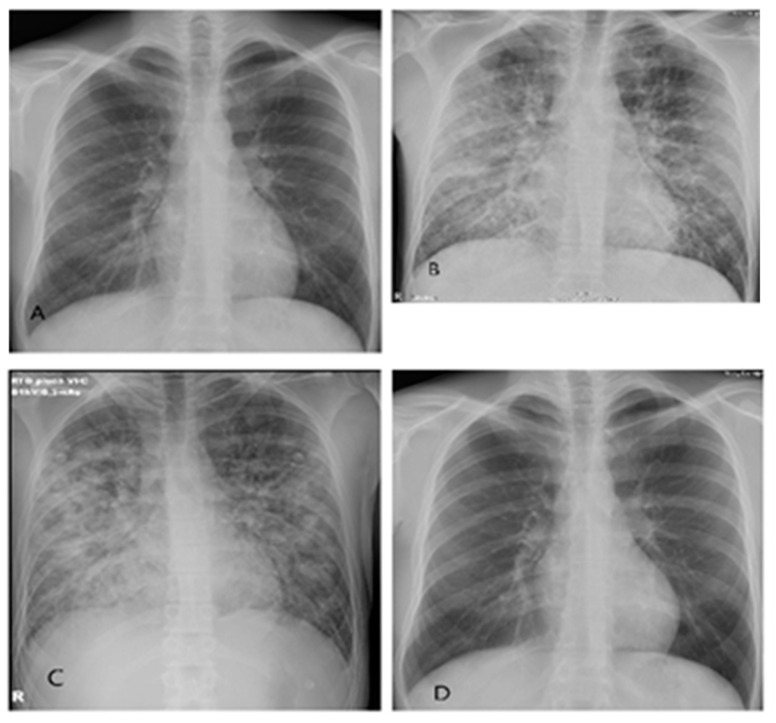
Series of thoracic X-rays in a patient with HPS caused by PUUV: (A) 30 October 2022, normal appearance of lungs and heart at admission. (B) 31 October 2022 (6 h interval), bilateral diffuse interstitial and alveolar infiltrates in all pulmonary fields. (C) 3 November 2022, very good regression of bilateral diffuse interstitial and alveolar infiltrates. Small bilateral basal pleural effusions. (D) 10 November 2022, complete regression of bilateral diffuse interstitial and alveolar infiltrates and pleural effusions.
